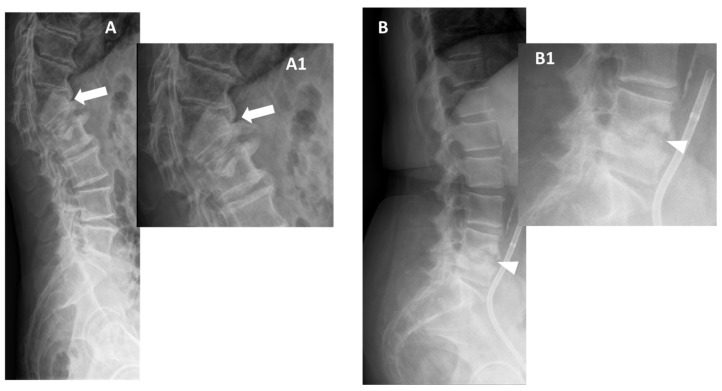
Conventional radiographs, lateral views (Panel A, and Magnification A1), of a 77-year-old male with previous pyogenic spondylodiscitis of T12-L1 vertebral bodies (partially collapsed and fused—arrows). Conventional radiographs, and lateral views (Panel B and Magnification B1) of a 64-year-old female with spondylodiscitis of L4–L5 vertebral bodies (thick endplate erosions are detected—arrowheads).
